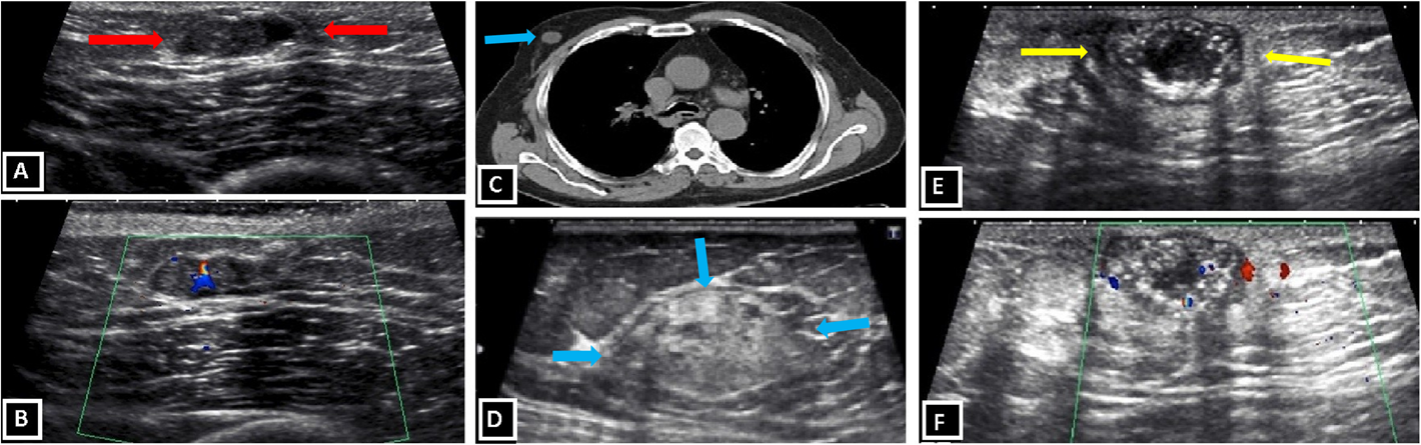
Benign neoplastic breast lesions. a, b Intraductal papilloma. Retroareolar well-defined solid nodule (red arrows) within a dilated duct is demonstrated in a 40-year-old patient with nipple discharge (a). Doppler ultrasound shows stalk of internal vascularity within the solid nodule (b). c, d Myofibroblastoma. Non-contrast CT scan displays well-defined round incidental mass (blue arrows) in right breast (c). US image shows circumscribed mass with mixed echogenicity and posterior acoustic shadowing due to internal fat content (d). e, f Pilomatricoma. Well-defined, lobulated complex mass with solid and cystic areas, hyperechoic spots, posterior acoustic shadowing (yellow arrows) and internal vascularity
